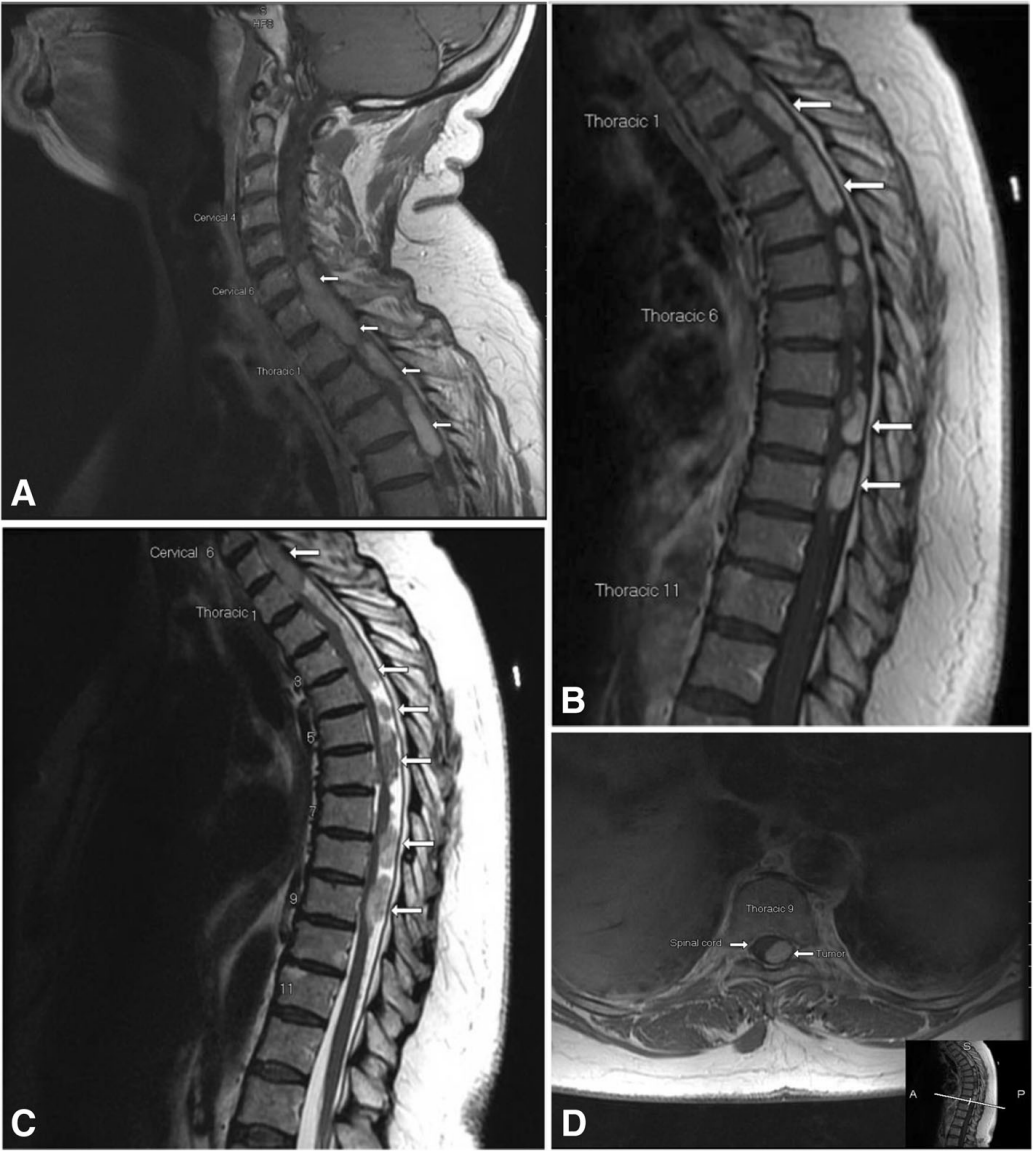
Magnetic resonance imaging of cervical - thoracic intradural extramedullary pilocytic astrocytoma. Initial MRI imaging of the cervical and thoracic spine, a Sagittal post contrast T1 cervical spine, b. Sagittal post contrast T1 thoracic spine, c. Sagittal T2 thoracic spine, demonstrates multiple enhancing intradural extramedullary tumors in the lower cervical and upper and middle thoracic spinal canal (white arrows indicate tumor). These cause multifocal severe spinal canal stenosis with marked flattening of the spinal cord, d. Axial post contrast T1 at level of T9
